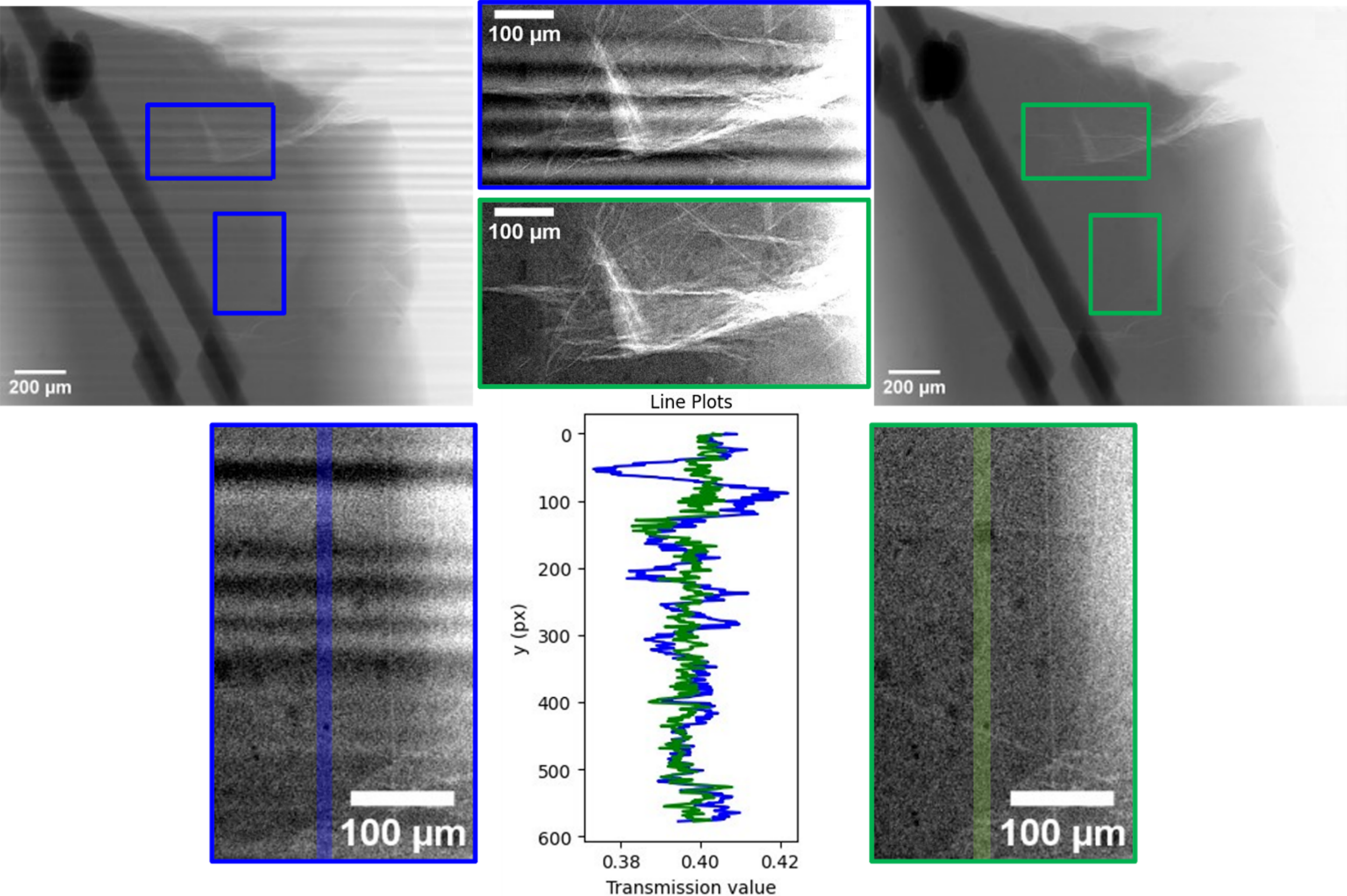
Radiographs of a daisy-chain structure, normalized using regular static flat-field correction (*a*, *c*, *e*) and multilayer-tilt normalization (*b*, *d*, *f*). 40 keV energy, 10× magnification (0.72 µm pixel size).
